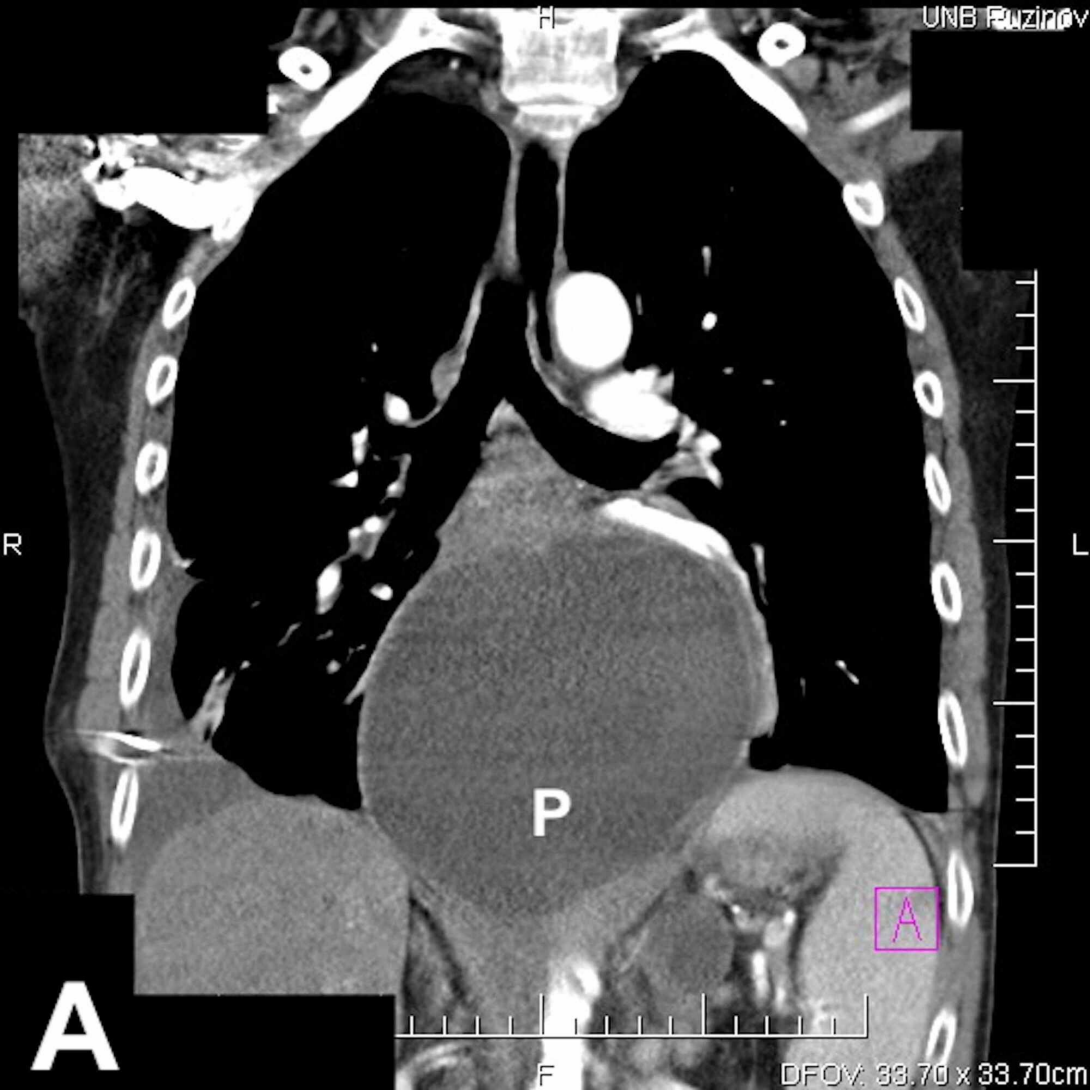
Chest CT scan (coronal) reveals a pseudocyst (P) extending from the body and the tail of the pancreas to the posterior mediastinum.
